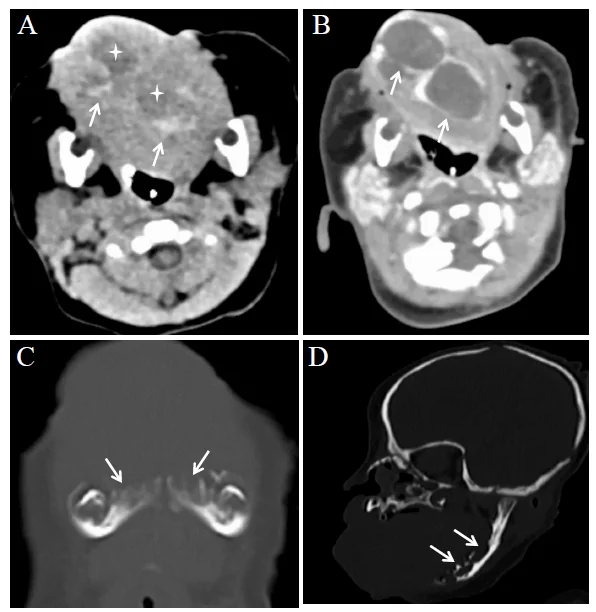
Postnatal MDCT at 2 days old. (A) Axial non-contrast CT shows an inhomogeneous mass with linear hyperdense areas (arrow) and multiple cystic hypodense areas (cross star). (B) Axial contrast-enhanced CT shows heterogeneous enhancement with rounded enhancing areas (arrow). (C, D) Axial and sagittal bone window images demonstrate bone resorption of the mandible adjacent to the mass (arrow).
